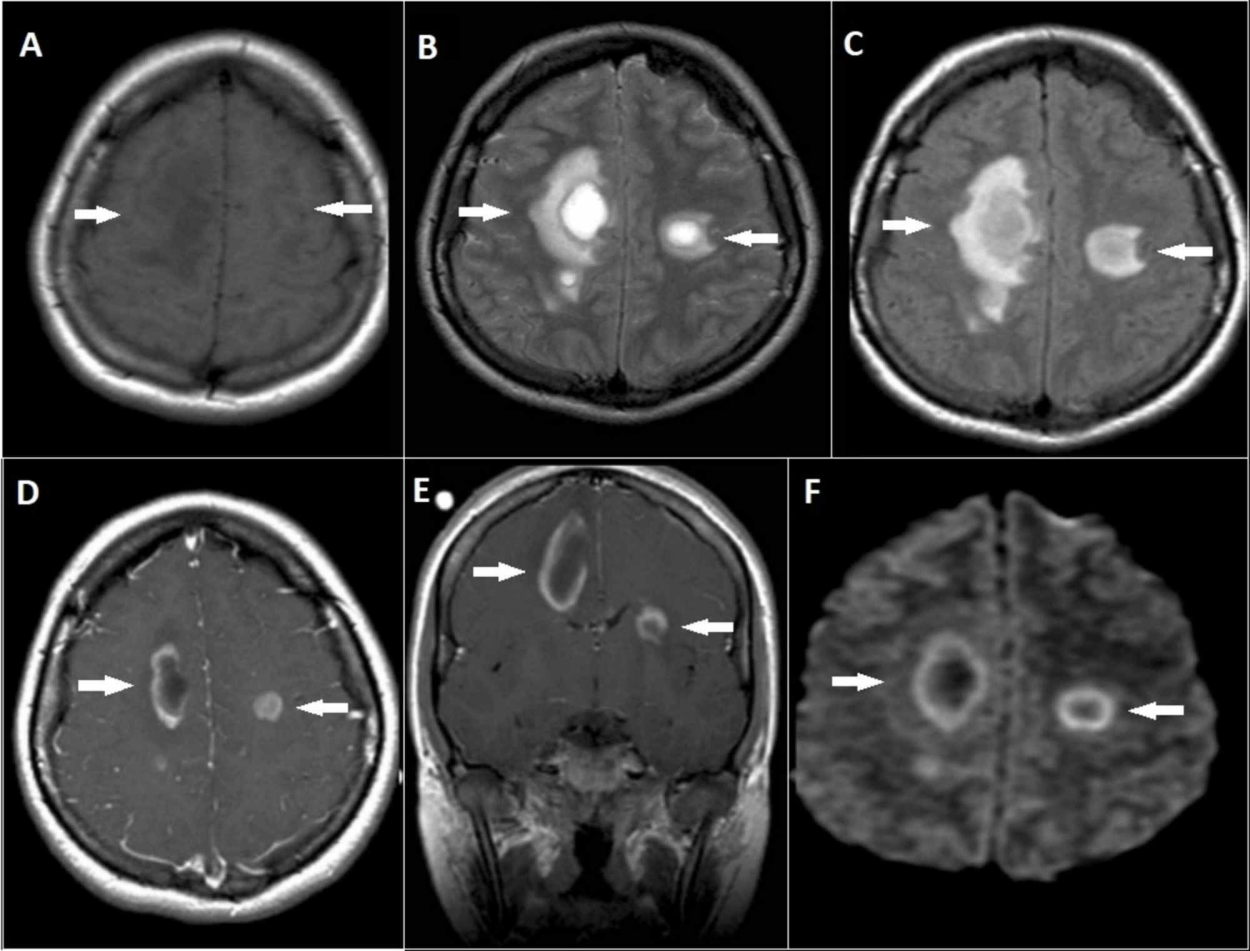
Magnetic resonance imaging of the head A - T1 axial scan showing hypointense areas in bilateral deep white matter at centrum semiovale and more marked on right side. B & C - Fluid-attenuated inversion recovery (FLAIR) and T2 axial scans showing hyperintense areas in bilateral deep white matter at centrum semiovale with perilesional edema and more marked on right side. D & E - Contrast-enhanced T1 axial and coronal scans showing open ring enhancement of the lesions. F - Diffusion-weighted 1 (DW1) axial scan showing peripheral diffusion restriction and no diffusion restriction in the center of the lesions.
